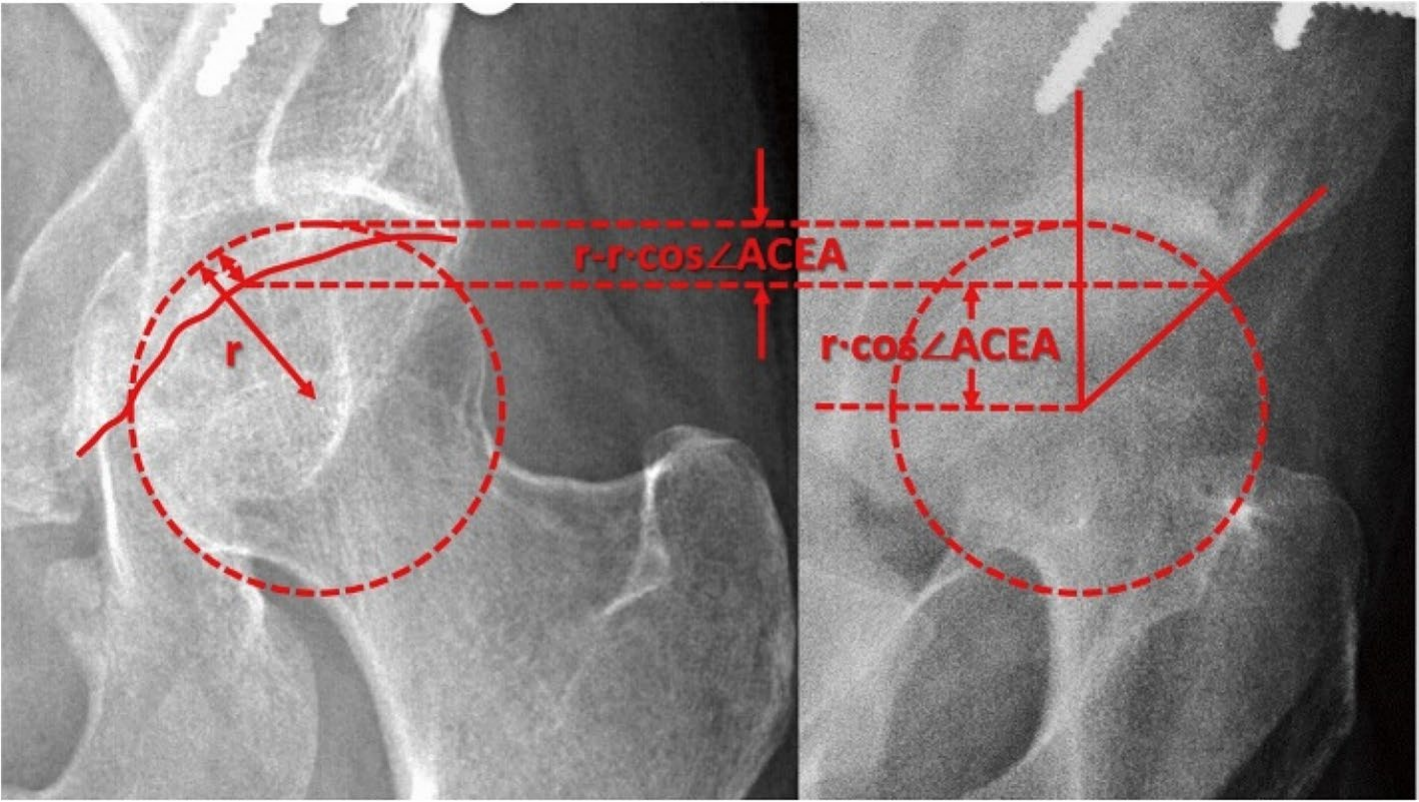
A rough conversion relationship of the AWI on a pelvic AP radiograph and the ACEA on an FP radiograph
